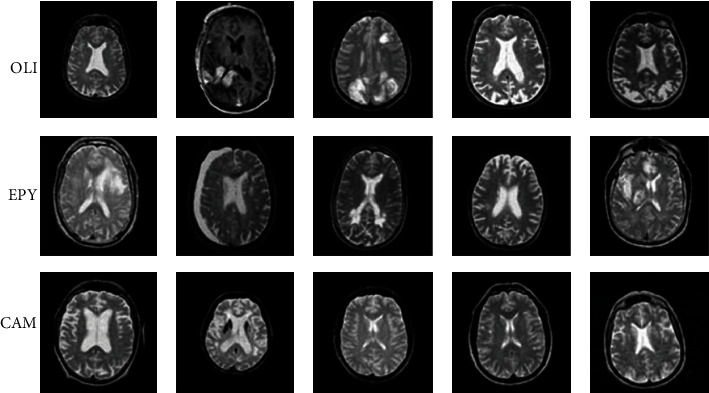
REMBRANDT dataset sample brain MR images.
